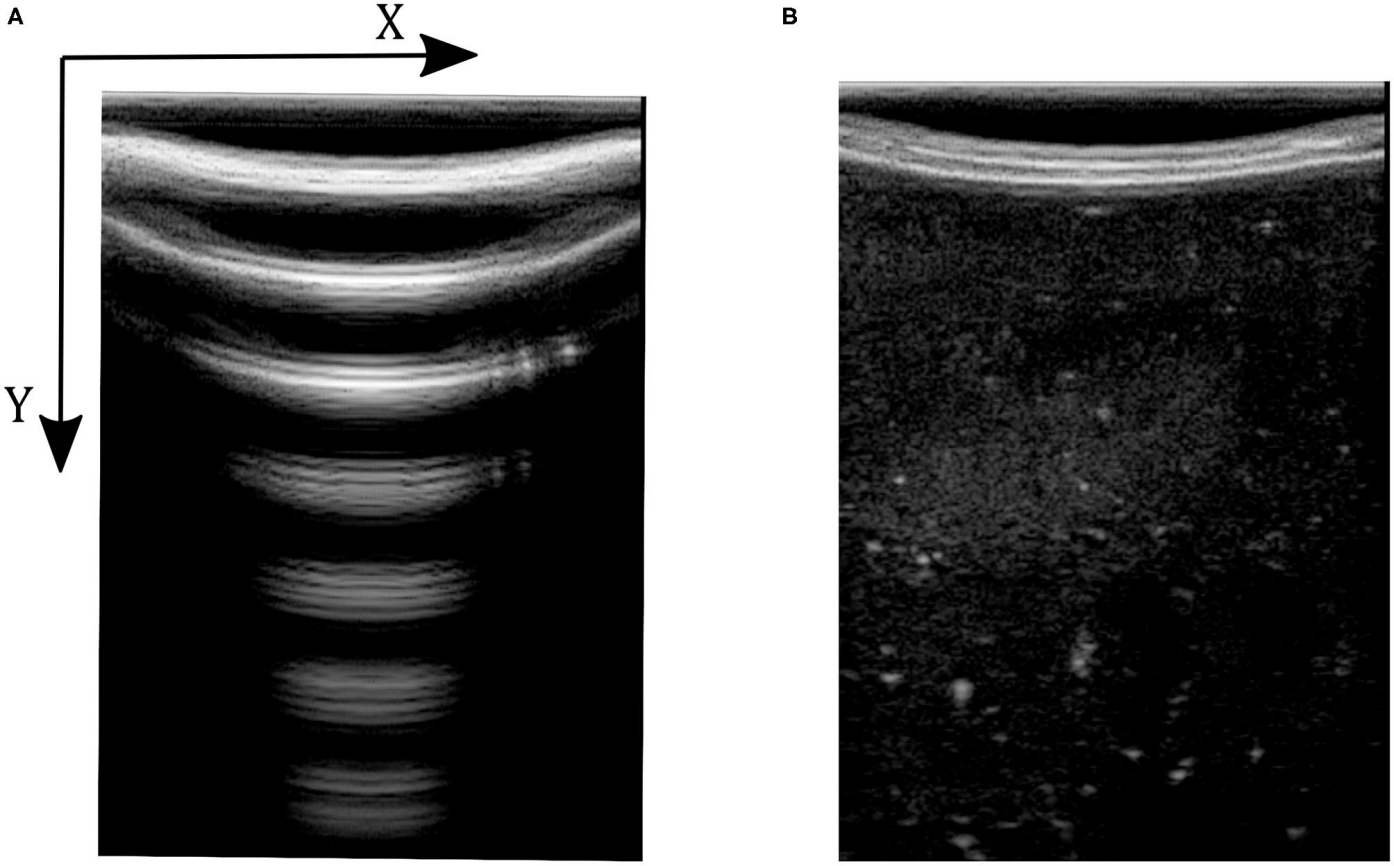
Ultrasound images with and without contact between tissue and probe. (A) Ultrasound image with no contact. (B) Ultrasound image with sufficient contact.
